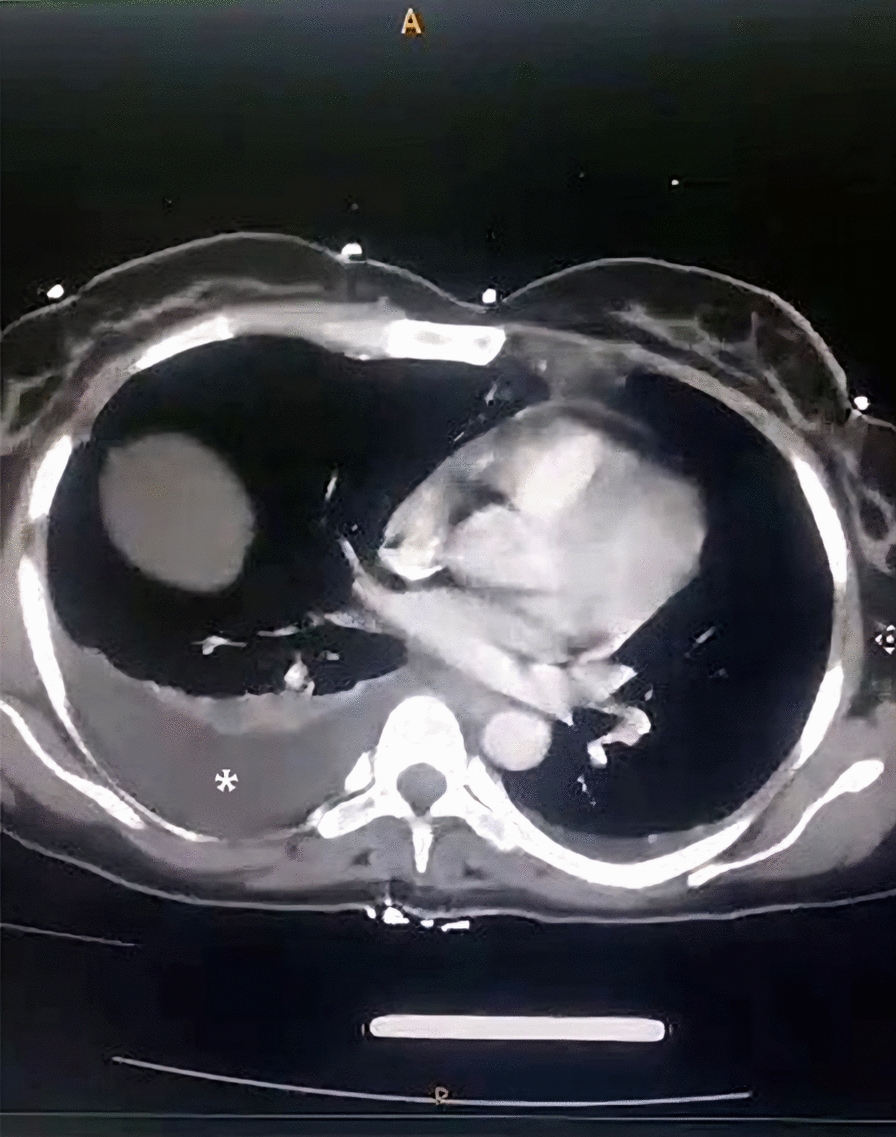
Axial computed tomography section of the chest showing large pleural effusion (white asterisks)
